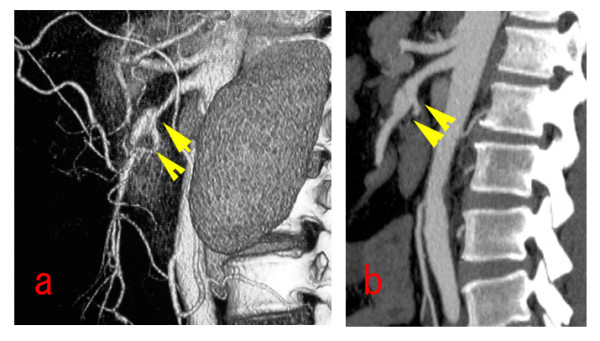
Sakamoto's type III dissection of the SMA. (a) preoperative three-dimensionally reconstructed images showing severe stenosis of the SMA with ULP, and the collateral flow from the celiac artery and inferior mesenteric artery. (b) postoperative 1 year abdominal enhanced CT scan show a thrombosed false lumen completely resolved without progressive dilation of ULP.
